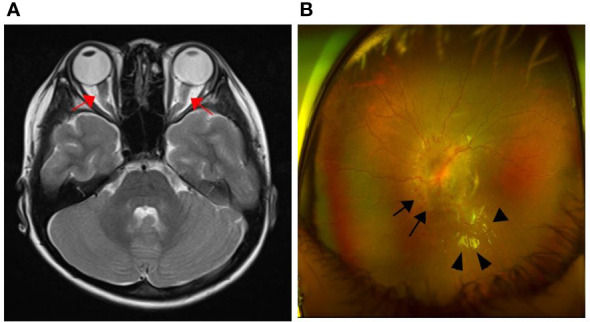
Brain MRI and fundoscopic findings at the time of visual disturbance onset. (A) Axial T2-weighted image showed diffuse swelling of both optic nerves (arrow); (B) Optic disc edema (arrow) and macular star (arrowhead).
